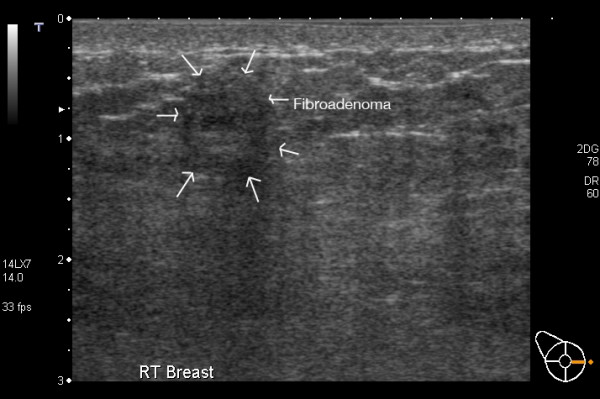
Fibroadenoma in a lactating breast. This fibroadenoma is difficult to image with ultrasound as it is almost the same echogenicity as the surrounding tissues.
